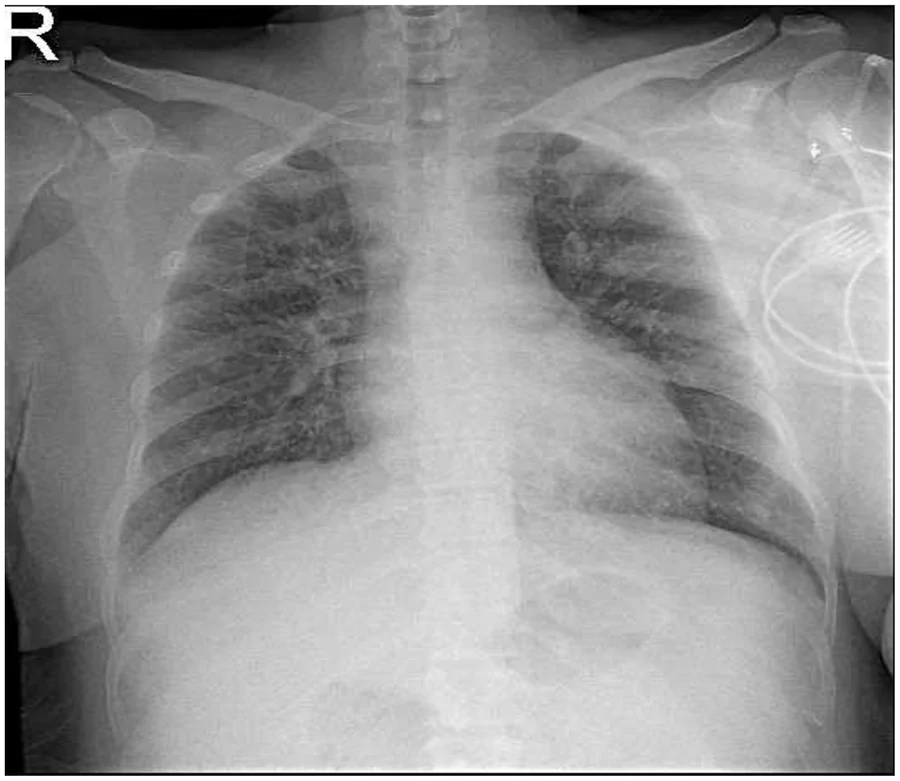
Chest radiography demonstrating bilateral pulmonary congestion and acute pulmonary edema on admission.
